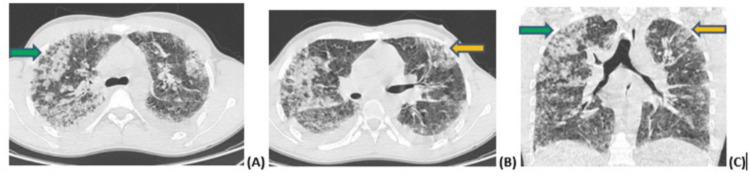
Figure 2 (A-C): CT scan in axial and coronal sections showing foci of condensation (green arrow) and ground-glass areas (yellow arrow), predominantly apical
